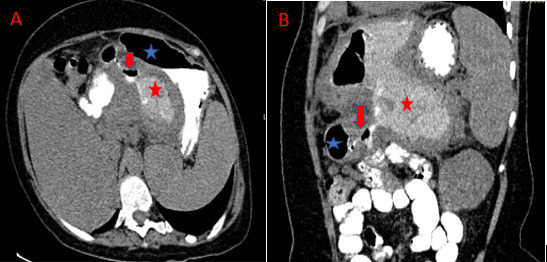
Abdominal CT after axial (A) and coronal (B)
